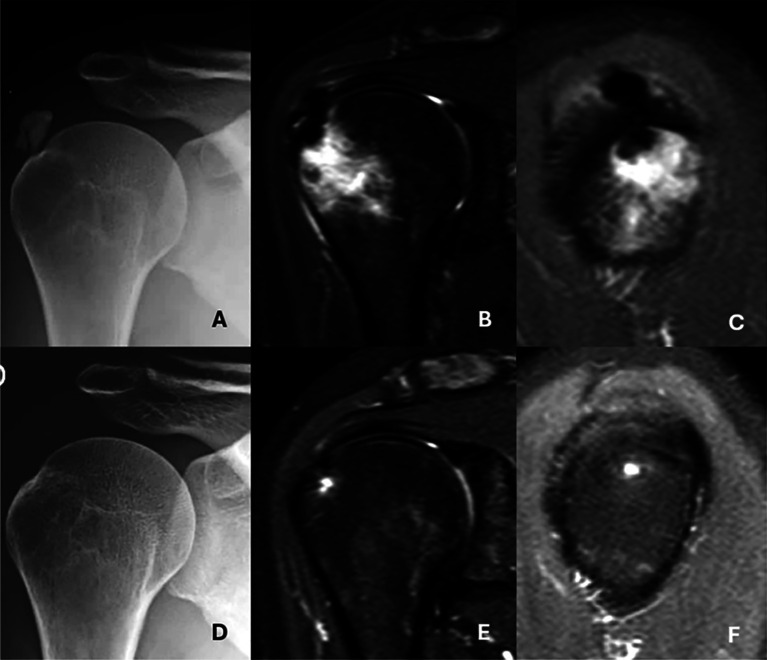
Advanced intraosseous migration (Type III-b) of a supraspinatus calcification with spontaneous resolution at follow-up.* A. Initial anteroposterior (AP) radiograph showing the calcific deposit. B. Coronal MRI revealing intraosseous extension of the deposit with associated bone marrow edema. C. Oblique sagittal MRI demonstrating the “hourglass sign,” indicating tendon-to-bone continuity. D. Follow-up AP radiograph two years later showing complete disappearance of the calcification. E. Coronal MRI confirming resolution of the intraosseous deposit. F. Oblique sagittal MRI showing complete resolution of both the calcification and bone marrow edema.
